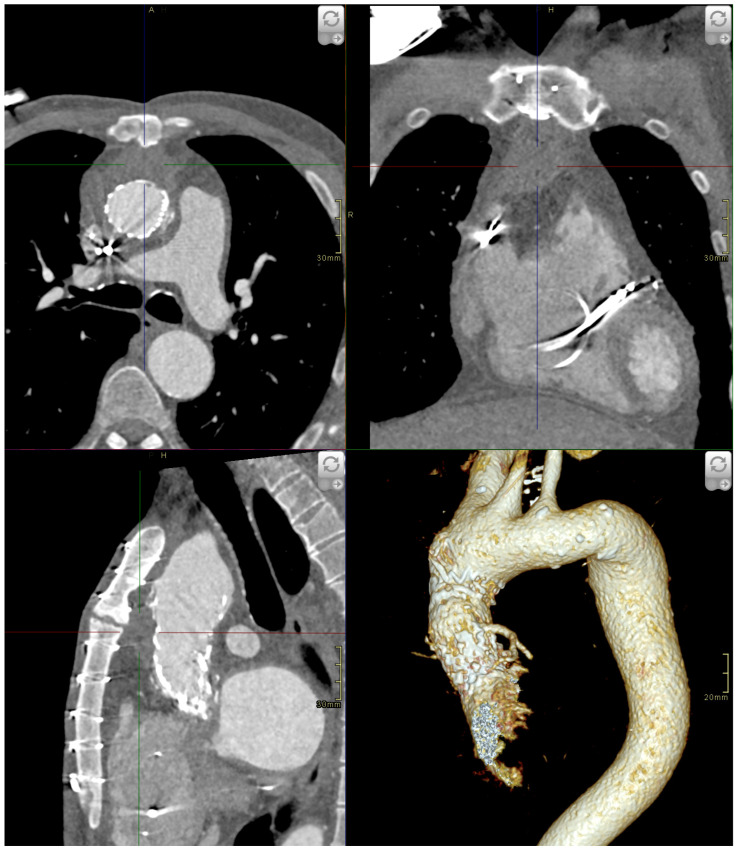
Two months’ follow-up CTA showing complete exclusion of the pseudoaneurysm. Clockwise from left bottom: sagittal plane arterial phase CTA, axial plane arterial phase CTA, coronal plane arterial phase CTA, volume rendering technique (VRT) 3D representation CTA.
